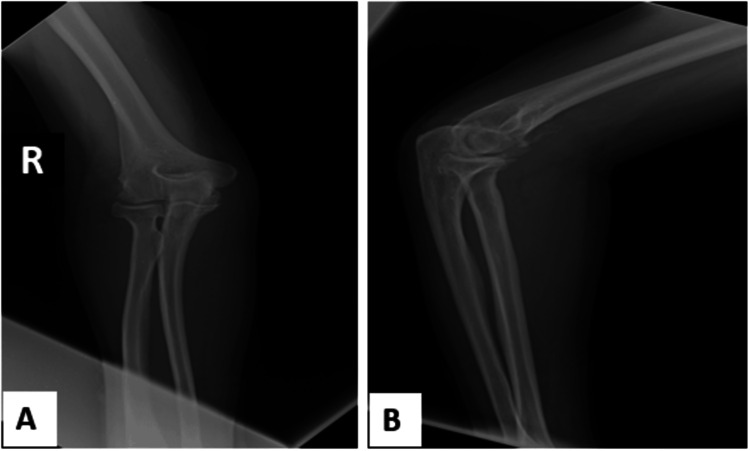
Postoperative radiographs of the right elbow (A) anteroposterior (B) lateral Shows no calcific densities.
